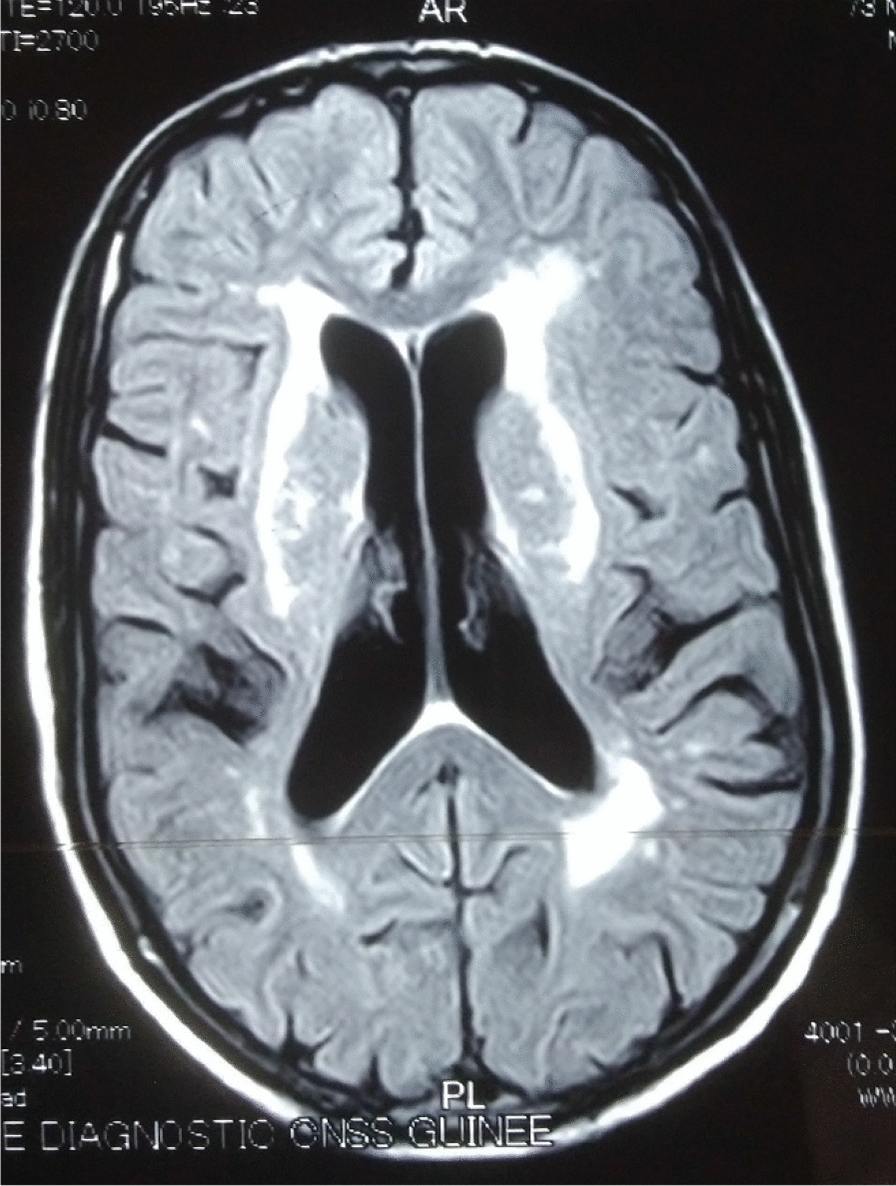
MRI cerebral: axial section FLAIR sequence abnormal white matter signal and cortical and subcortical atrophy
